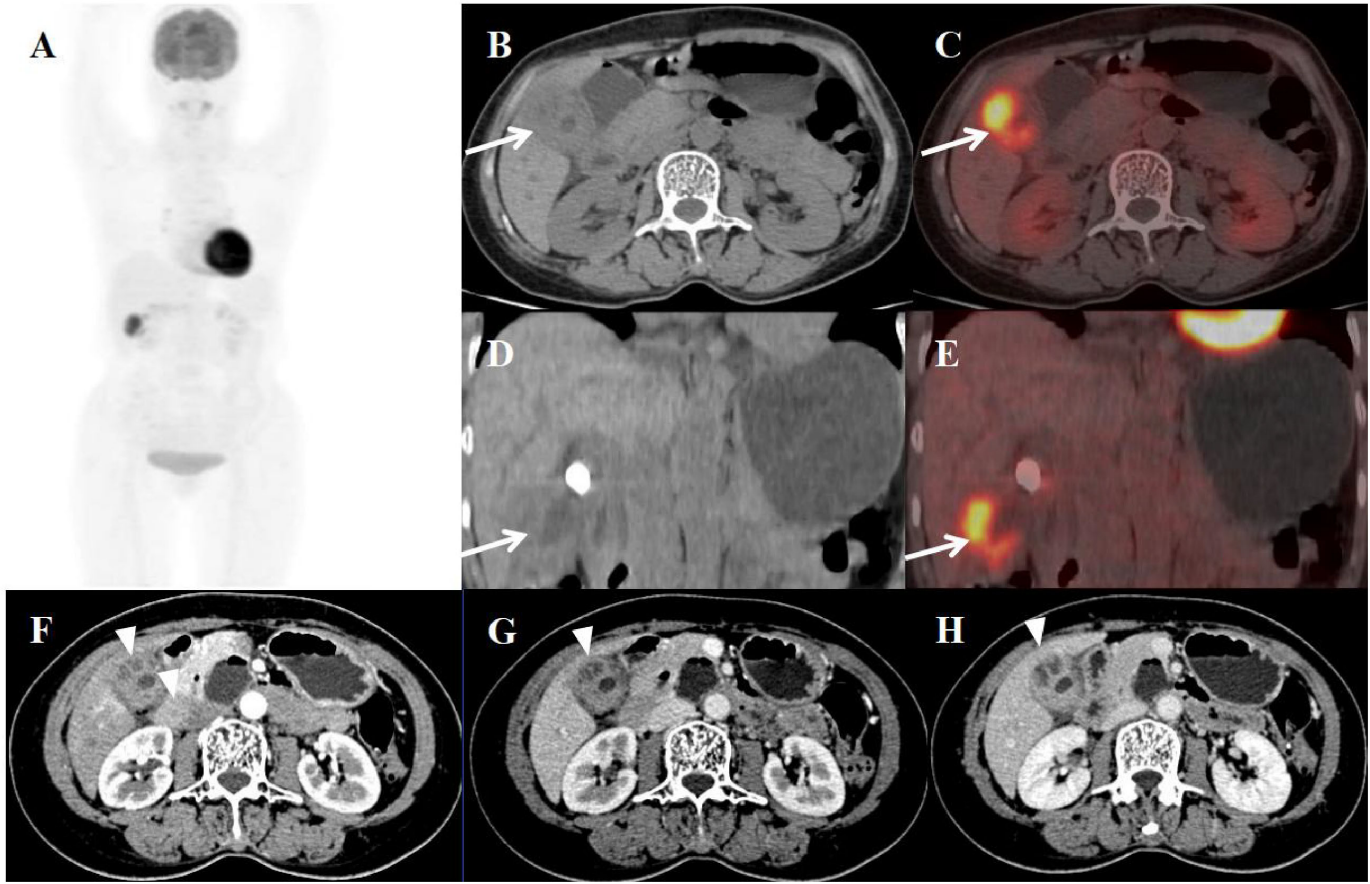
Female, 58 years old. (A) (whole body MIP), (B, C) (Axial CT and fused axial PET-CT), (D, E) (Coronal CT and fused coronal PET-CT), and (F–H) (axial, multiphasic contrast-enhanced CT). 18F-FDG PET/CT Imaging Findings: The gallbladder lumen was narrowed with impacted calculi (D). Focal thickening of the gallbladder wall was present, most prominent at the fundus (approximately 20 mm) (B, D, arrow ↑), accompanied by hypodense intramural nodules. There is markedly increased FDG avidity, with an SUVmax of 10.6 (C, E, arrow ↑). The hepatobiliary interface is indistinct, though the gallbladder mucosal line remains continuous, and no enlarged lymph nodes are visualized in the surrounding region. Multiphase contrast-enhanced CT reveals progressive delayed enhancement of the thickened gallbladder wall, with ring enhancement of the wall nodules observed in the delayed phase (H, arrow ▲) (CT values: 44 HU non-contrast, 67 HU arterial, 76 HU venous, 83 HU delayed).
